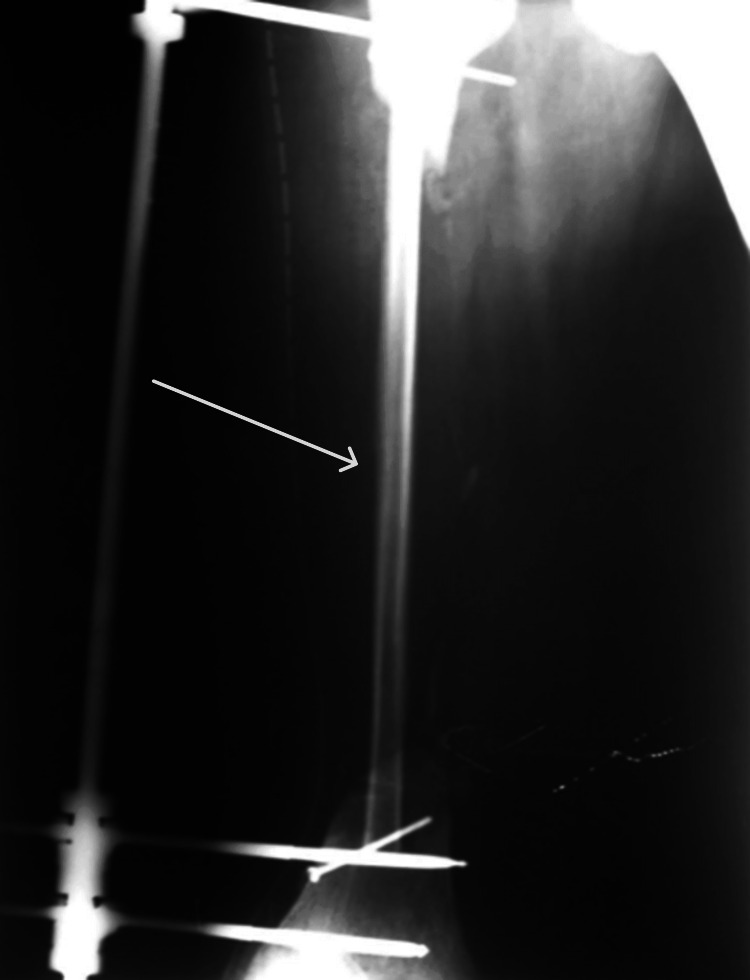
X-ray image taken 2 weeks postsurgery showing a thin fibula. The thin fibula is indicated by the arrow.
